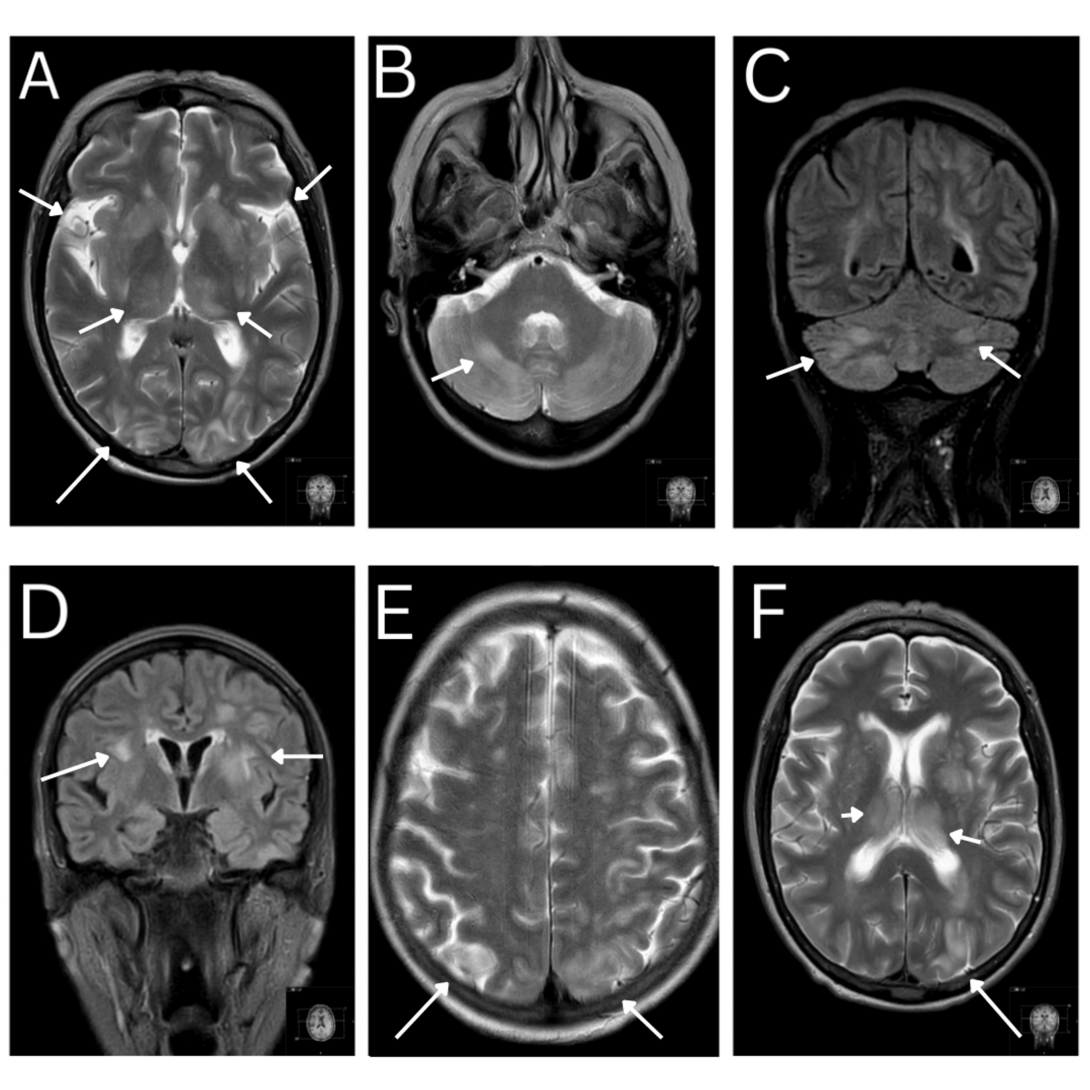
High T2 signals within cerebrum and cerebellum consistent with posterior reversible encephalopathy syndrome (PRES) A: Axial MRI head showing high T2 signal within the subcortical white matter of basal ganglia, both occipital lobes and thalami B: Axial MRI head showing high T2 signal within the subcortical white matter of cerebellar hemispheres C: Coronal MRI head showing high T2 fluid-attenuated inversion recovery (FLAIR) signals throughout cerebellum D: Coronal MRI head showing high T2 FLAIR signal within the subcortical white matter of left inferior temporal lobe and basal ganglia E: Axial MRI head showing posterior high T2 signal within occipital lobe F: Axial MRI head showing high T2 signal within the left parietal lobe, basal ganglia and thalami
